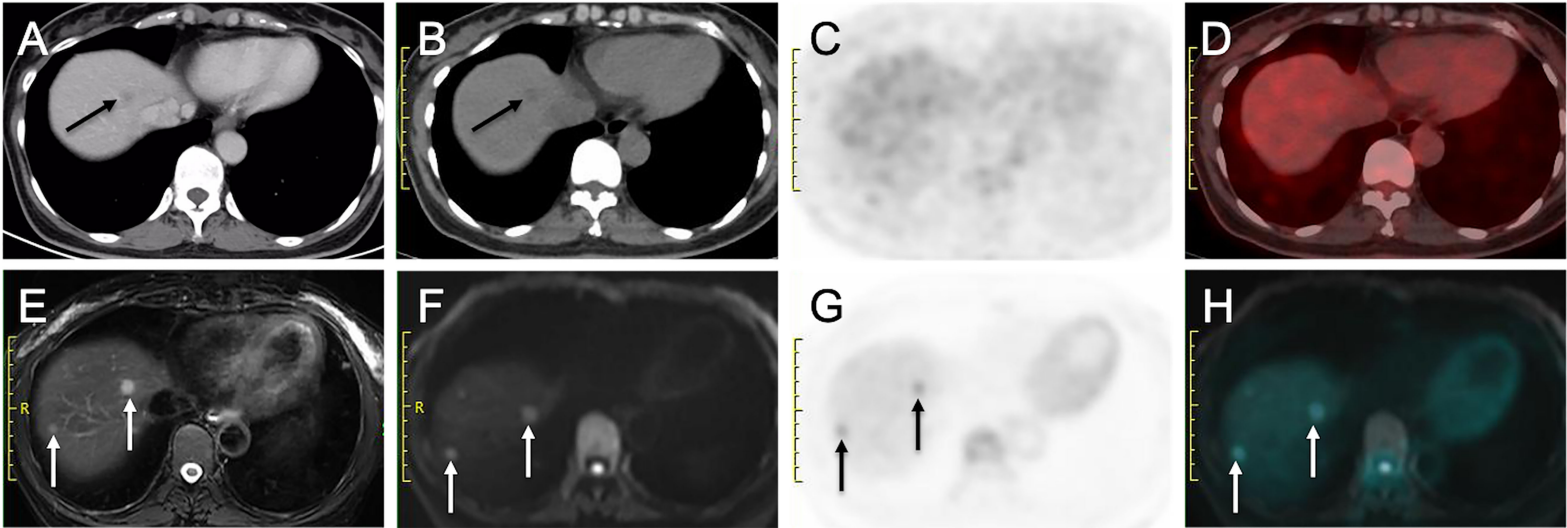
More liver metastases detected on delayed PET/MRI than on CE-CT and early PET/CT. (A) Venous phase CT image shows a hypo-enhanced nodule in liver (arrow). (B) Nonenhanced CT image shows a hypoattenuating nodule in liver (arrow), (C) Early PET image and (D) Corresponding PET/CT fusion image show that the nodule does not show obvious hypermetabolism. (E) Fat-suppressed T2WI shows two hyperintense nodules in liver (arrows). (F) DW image (b = 800 sec/mm2) shows two nodules with restricted diffusion (arrows). (G) Delayed PET image shows two hypermetabolic nodules in liver (arrows). (H) Corresponding PET and DW fusion image shows the two nodules with both hyperintense and hypermetabolism (arrows).
